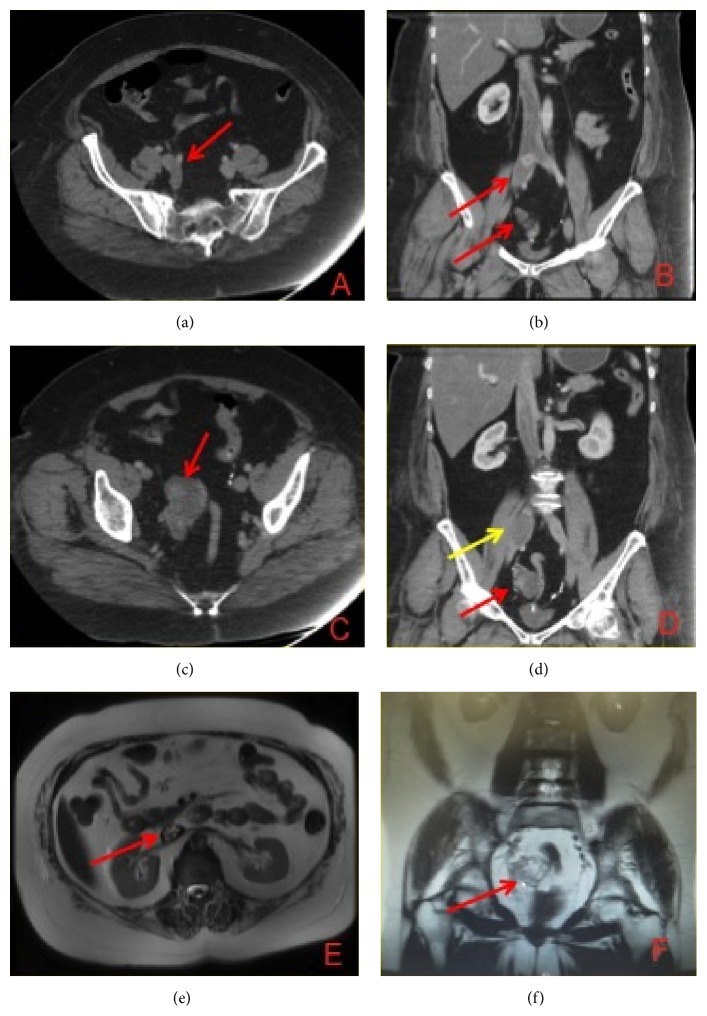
(a) CT axial and (b) coronal views of the IVL in the right common iliac vein (red arrows). (c) Axial and (d) coronal views of the pelvic component of the tumor (red arrows), abutting the dilated common iliac vein (yellow arrow). (e) Axial T2-weighted sequence on MRI identifying the intraluminal caval tumor with (f) associated pelvic mass on coronal image.
